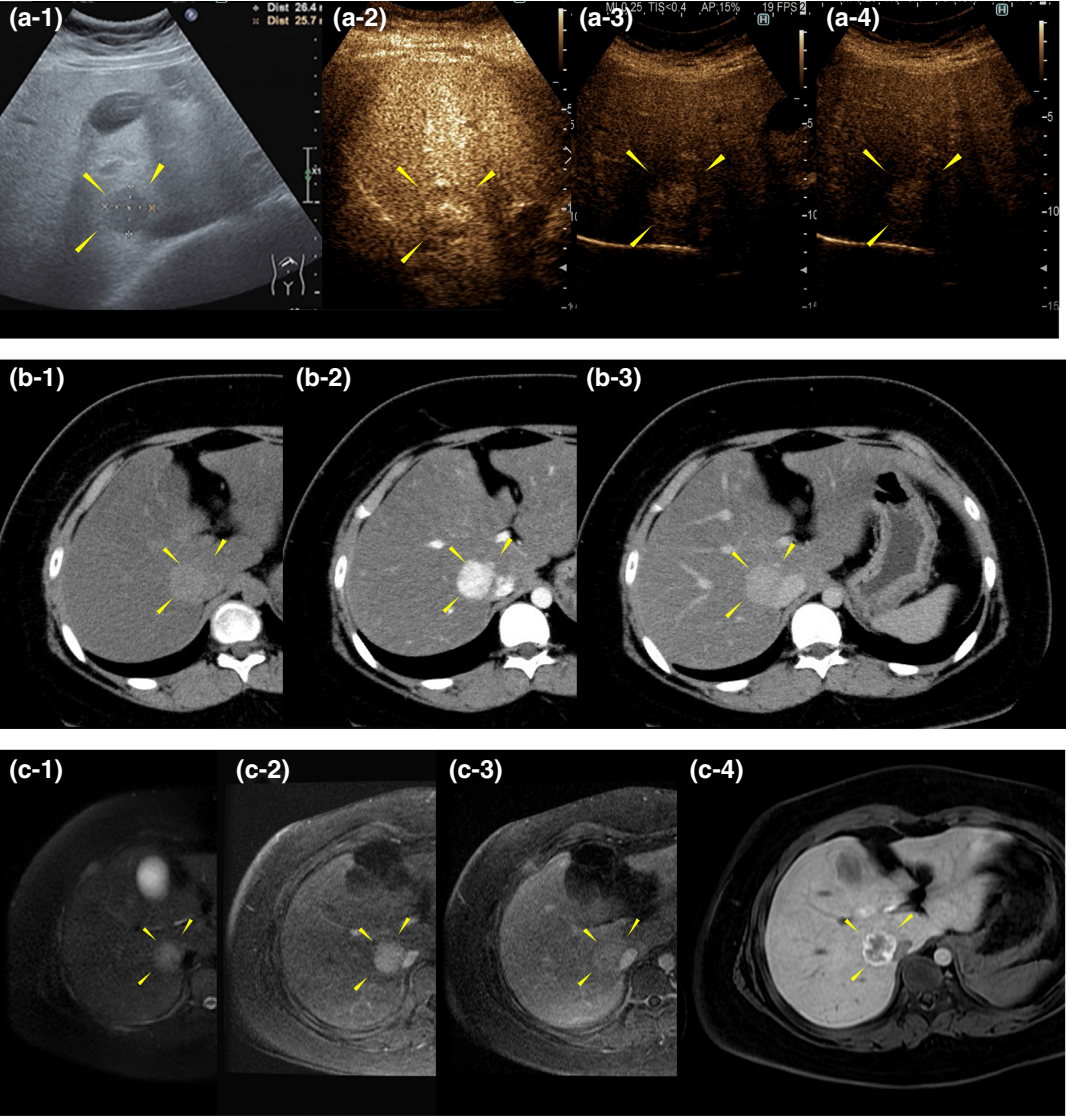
Liver imaging findings. (a) Ultrasonographic images. a‐1, B‐mode; a‐2, arterial phase; a‐3, portal vein phase; a‐4, post vascular phase in harmonic tissue by perflubutane contrast‐enhanced imaging. (b) Enhanced computed tomography images. b‐1, nonenhanced; b‐2, arterial phase; b‐3, late phase. (c) Gadoxetate disodium‐methoxybenzyl‐diethylenetriamine pentaacetic acid contrast‐enhanced magnetic resonance images. c‐1, nonenhanced T2‐weighted; c‐2, arterial phase; c‐3, portal vein phase; c‐4, hepato‐biliary phase. The yellow marks indicate the FNH‐like lesion.
